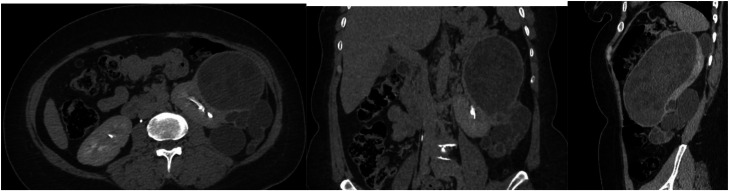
Delayed phase contrast-enhanced CT): multiplanar reconstructions (axial, coronal, sagittal) demonstrate: no communication with the ipsilateral collecting system.
